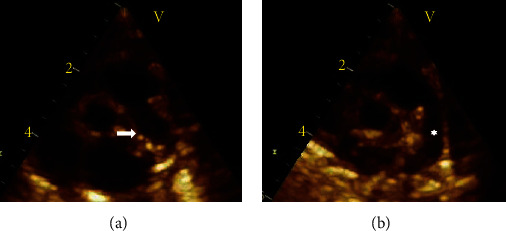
Cross-sectional echocardiogram (parasternal short axis view) showing (a) the LPA at its typical location (arrow) and absence of the RPA and (b) large left side PDA (asterisk). LPA: left pulmonary artery. RPA: right pulmonary artery.
